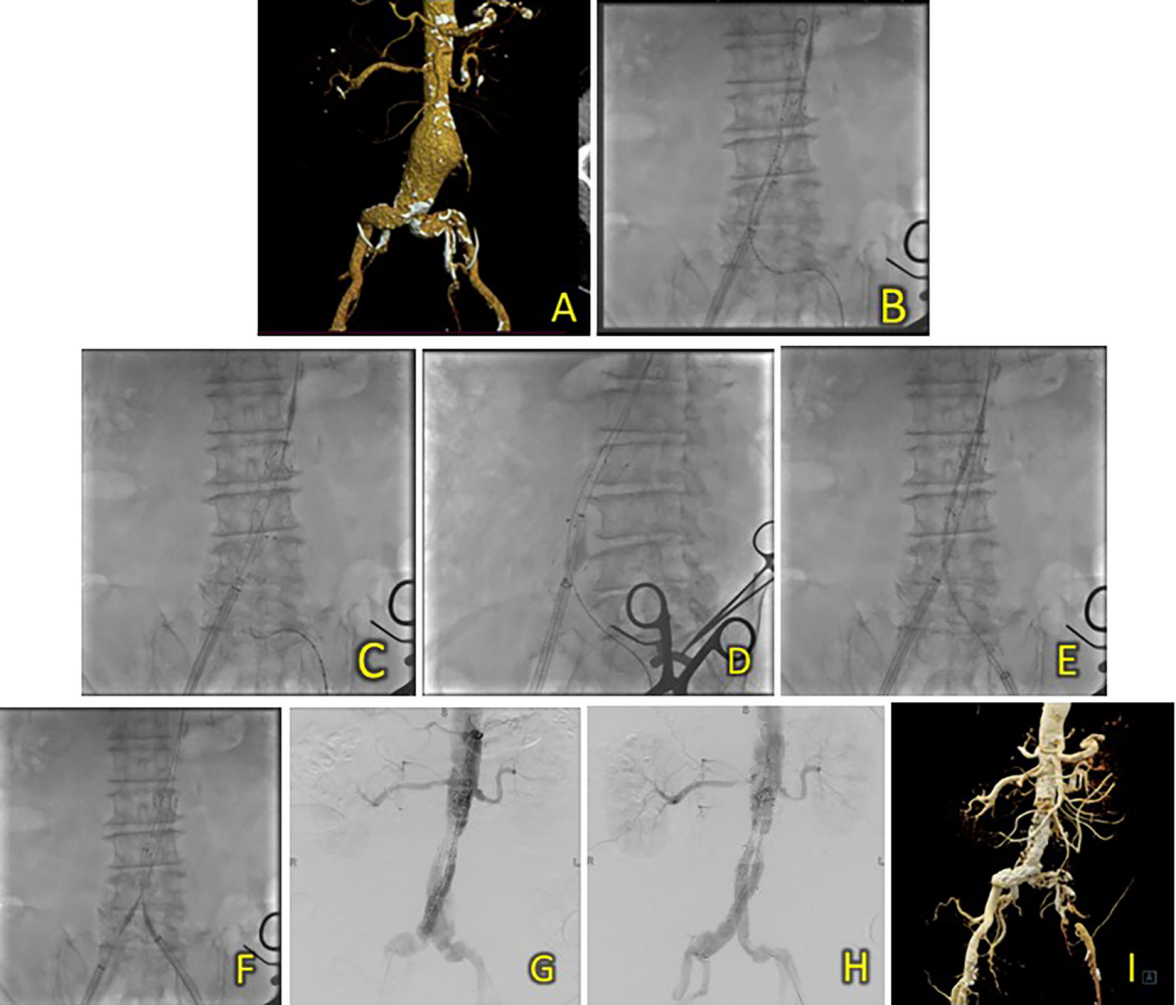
T&K B-EVAR on Patient 1. (A) CT of the 1
